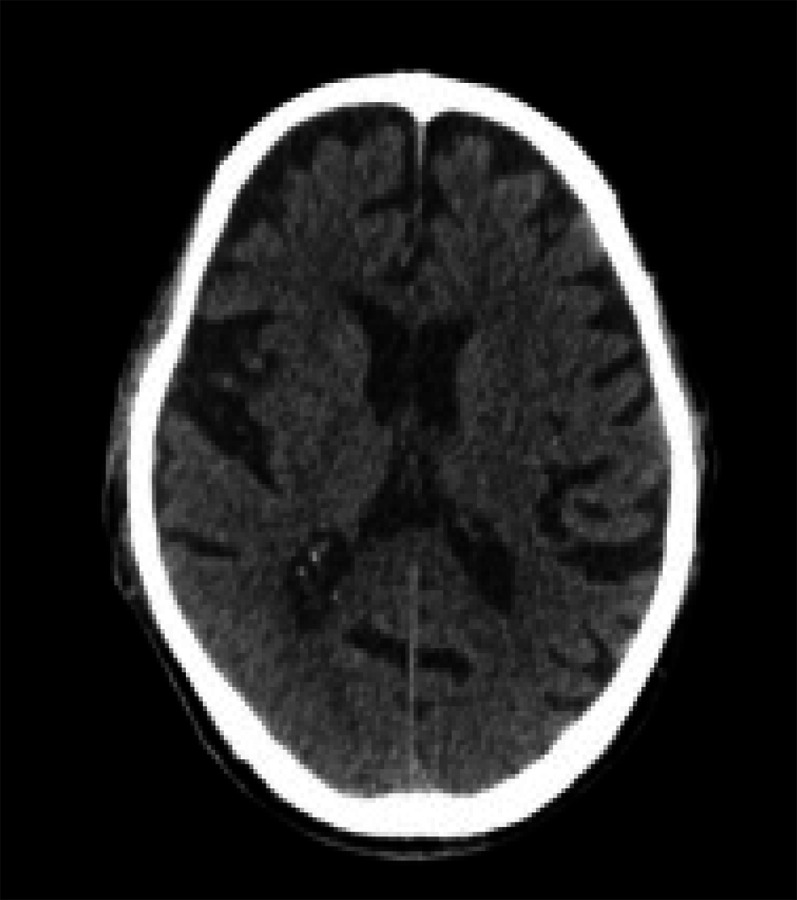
CT scan of the head showing a normal scan with cerebral atrophy.
